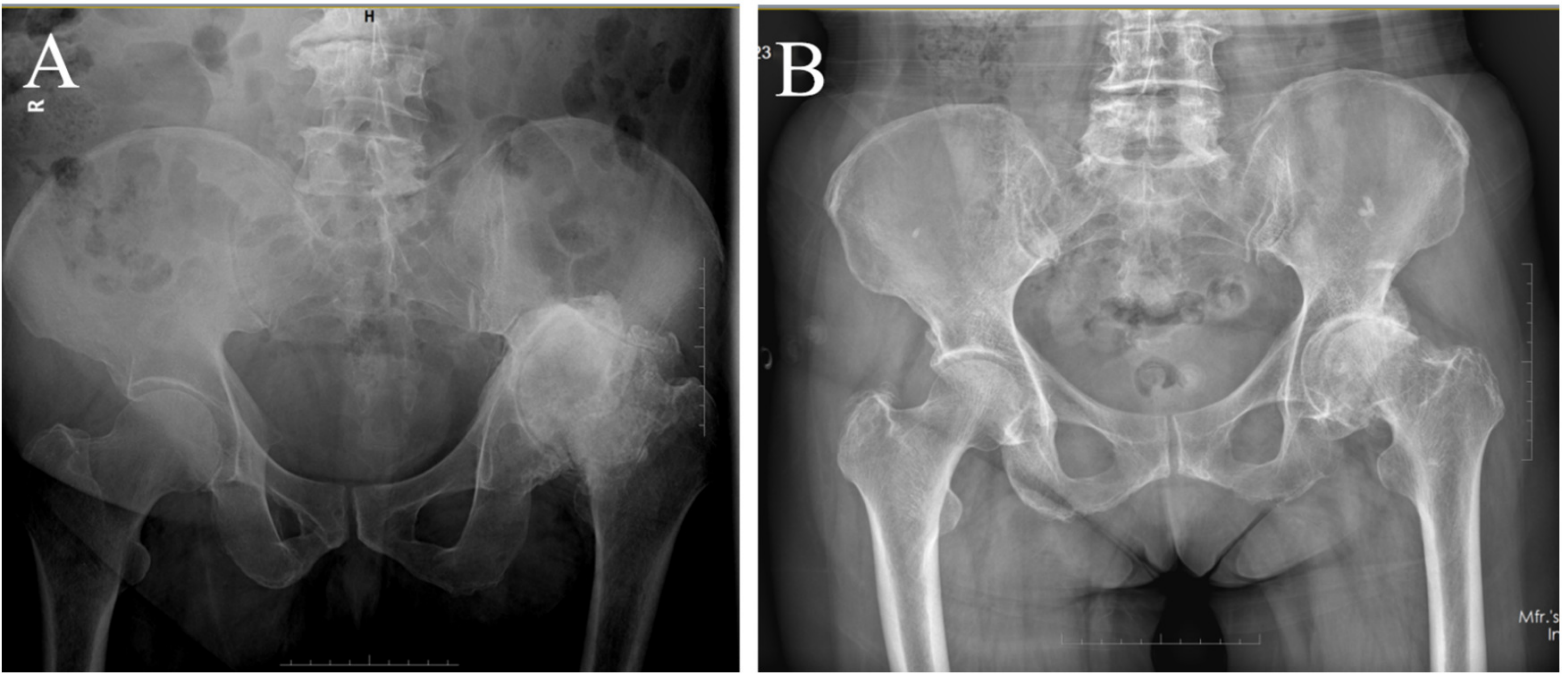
Long-term unreduced hip joint dislocation, arthritis of the hip on the anteroposterior radiograph of the pelvis. (A) Rotational center shift upward and outward, image of the real acetabulum and aureole sign can be observed simultaneously on images of long-term unreduced hip joint dislocation. (B) On images of osteoarthritis of the hip, there is no upward migration or exteriorization of the center of rotation.
